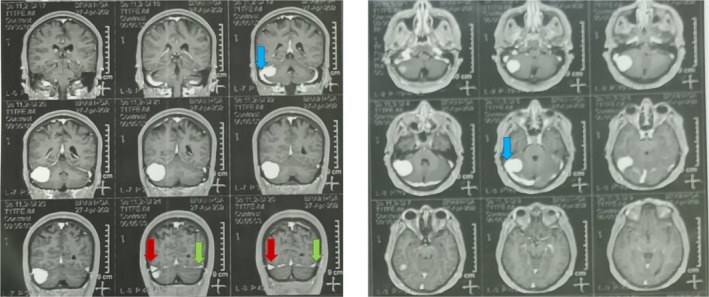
Preoperative brain MRI with Gad: There is an avidly enhancing lesion in the transverse and sigmoid sinus junction (blue arrows). The right transverse sinus (red arrow) is much more prominent than the left one (green arrow), indicating the right dominant venous sinus system.
